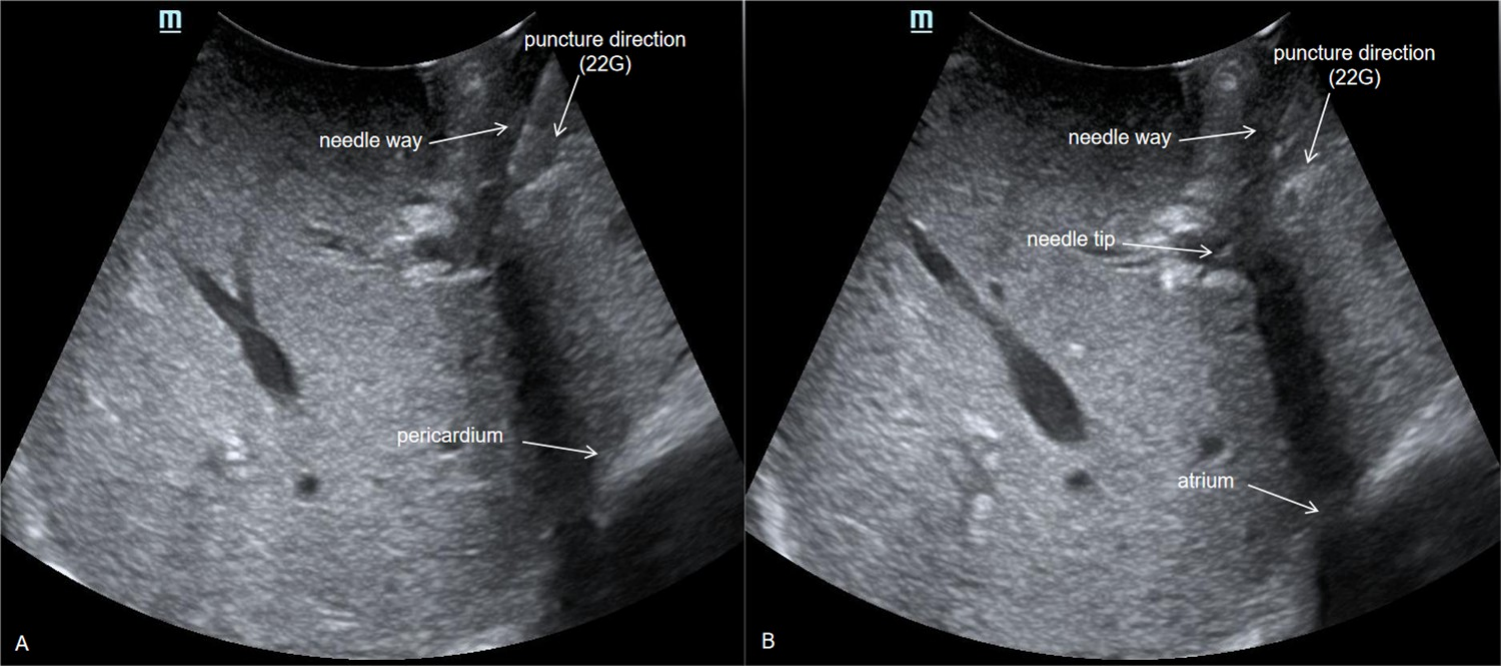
Intraoperative ultrasound-guided PVP measurement image.
